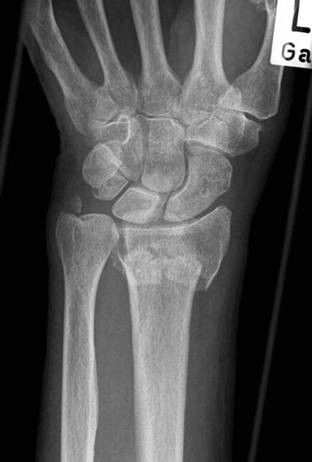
Intraarticular distal radius fracture. Anteroposterior radiograph of an intraarticular distal radius fracture type C1-AO/ASIF
